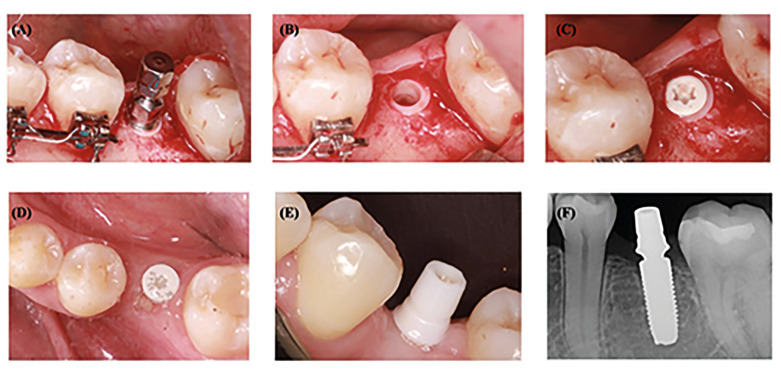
Clinical procedure for T-PZI. (a) implant placement with driver tool. (b) Bone-level implant situation. (c) Implant with cover screw. (d) Implant after the osseointegration period with healing abutment. (e) Implant with definitive abutment (f) Periapical radiograph. Courtesy of Dr. Jorge Cortés-Bretón Brinkmann, Madrid.
